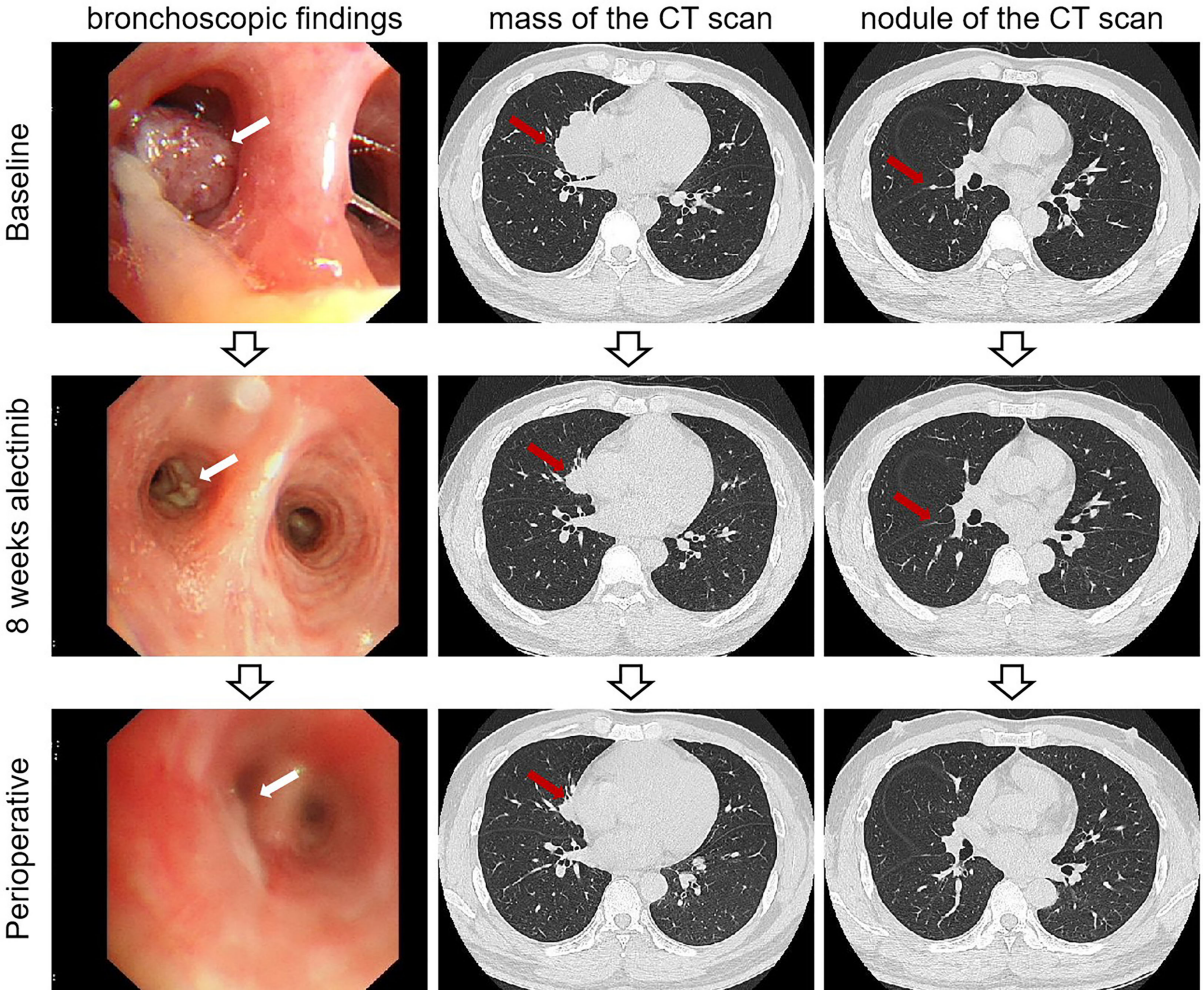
Imaging findings of case 2. The bronchoscopic findings and computed tomography scan of baseline and after neoadjuvant alectinib. CT, computed tomography.
